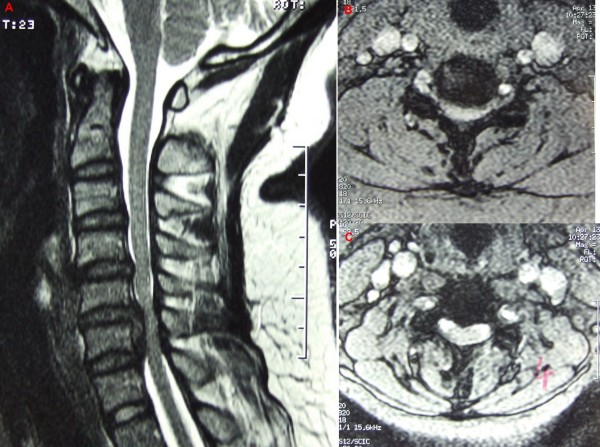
Preoperative MRI image showing compression of the cervical cord at C5/6. A, sagittal T2-weighted MRI image. B, corresponding axial T2-weighted MRI image showing deformation of the C5/6 by the compression. C, no deformation of the cord at C4/5.
